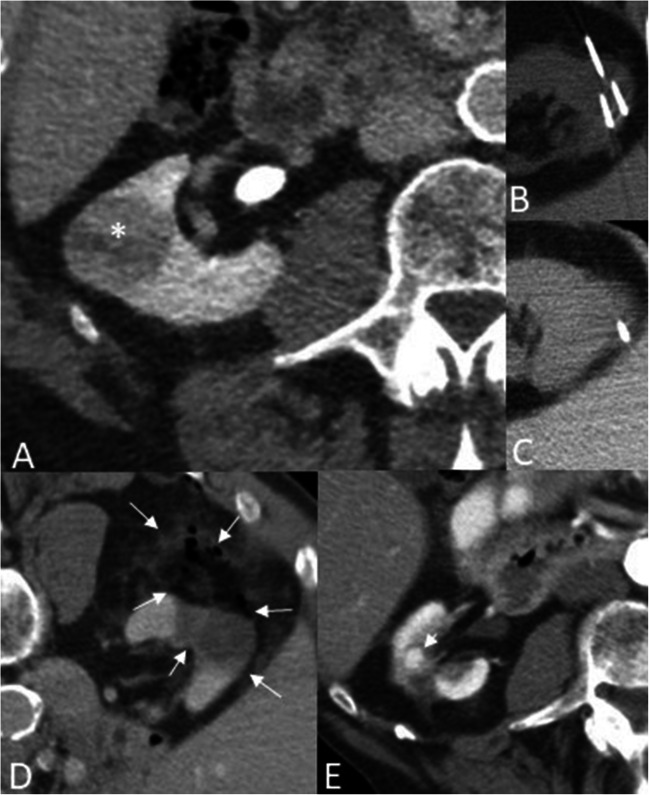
A RCC treated by RFA. A pretreatment contrast-enhanced CT image with a RCC in the right kidney is shown (* in panel A). In panel B and C needle placement in consecutive images during RFA is shown. Three RFA needles are placed through the tumor. Note that the RFA procedure was performed with the patient in a prone position. Panel D shows an image directly after ablation. The ablation zone is marked by arrows. Ablation was considered successful; however, 1 year later, a local recurrence was noted (arrow in panel E, patient in supine position). The recurrence was successfully treated with a second RFA procedure
